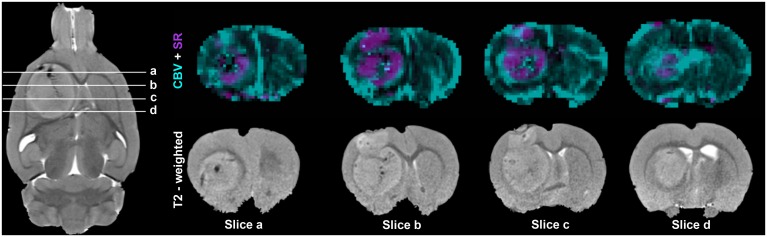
Spatial heterogeneity of cerebral blood volume (CBV) and signal recovery (SR). Maps of SR (purple) were overlaid on maps of CBV (green) obtained on day 21 after injection of 100,000 C6 cells. Areas of increased CBV were mostly found at the tumor rim whereas signal recovery exceeding the baseline was mainly seen in the tumor center excluding regions which were most likely necrotic (dark on T2 weighted images). Coronally and axially oriented T2-weighted images (T2w) are shown as reference.
